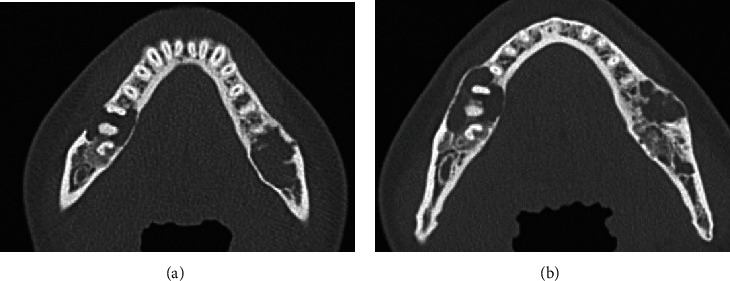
Follow-up CT images. (a) Three months and (b) twenty-seven months after the incisional biopsy; the CT images indicate enlargement of the right and an anterior half area of the left low-density lesions. CT: computed tomography.
